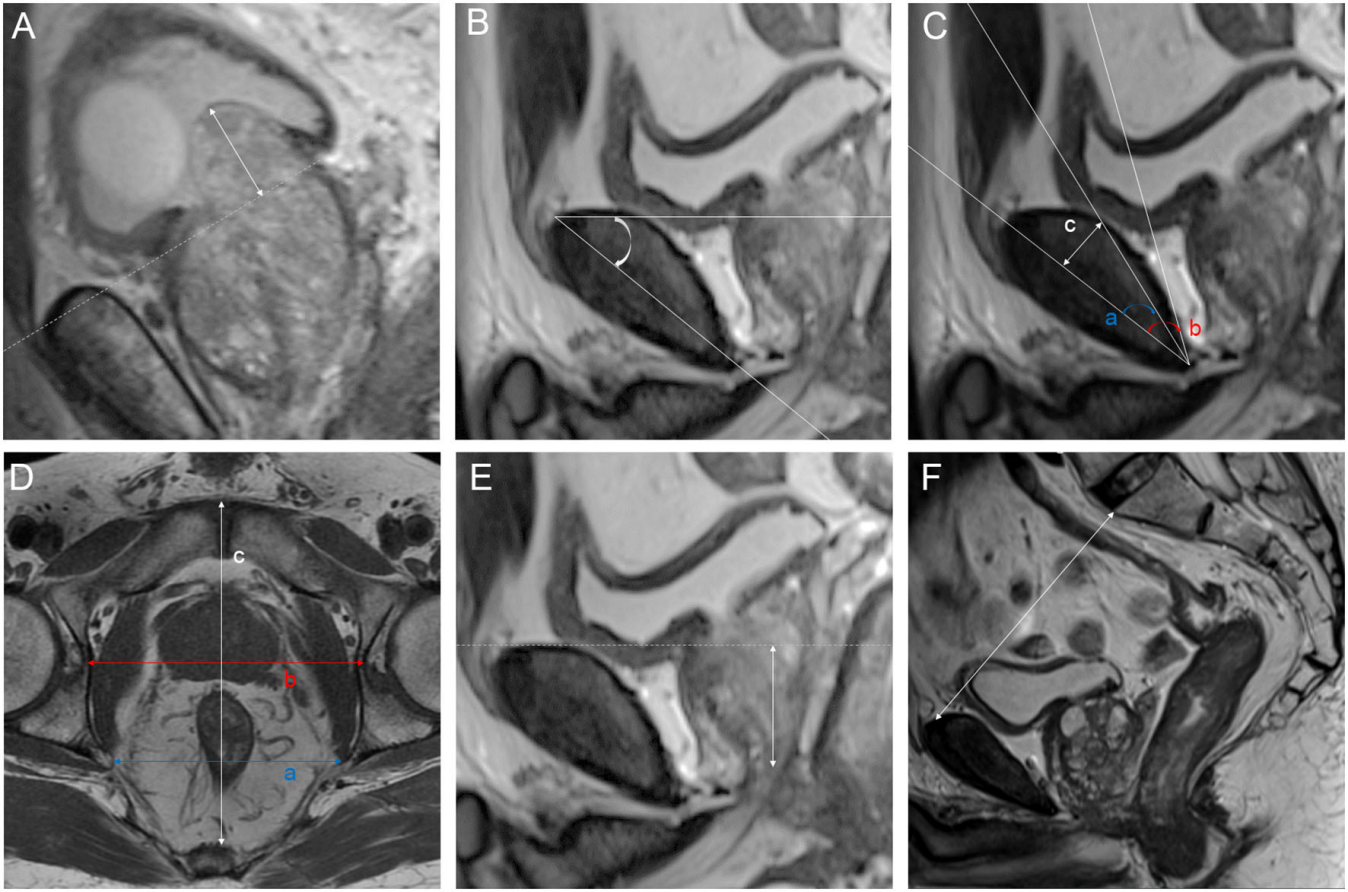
Parameters measured on magnetic resonance imaging: (A) bladder protrusion: the distance from the most prominent point of the prostate in the bladder to the outer boundary of the bladder wall; (B) symphysis angle: the angle between the long axis of the symphysis pubis and the horizontal line on the mid-sagittal image; (C) a: pubic angle 1: the angle between the line between the most inferior point of the pubic bone to the most bulging point of the posterior cortex and the pubic axis; b: pubic angle 2: the angle between the most inferior curve of the pubic bone and pubic axis; c: curve distance: the vertical distance from the most bulging point of the posterior cortex of the pubic bone to pubic axis; (D) a: interspinous distance (ISD): the distance between the bilateral ischial spines; b: bony width of the pelvis at the mid-femoral head level (BFW); c: work space AP: the distance from the anterior peritoneum to the anterior border of the coccyx (inner border); (E) apical depth (AD): the vertical distance from the distal margin of the prostatic apex to the horizontal line of the most proximal edge of the pubic symphysis on the midsagittal image; (F) pelvic brim distance: the distance from the median point of the upper margin of the pubic symphysis to the median point of the upper margin of the sacral promontory on the midsagittal image.
